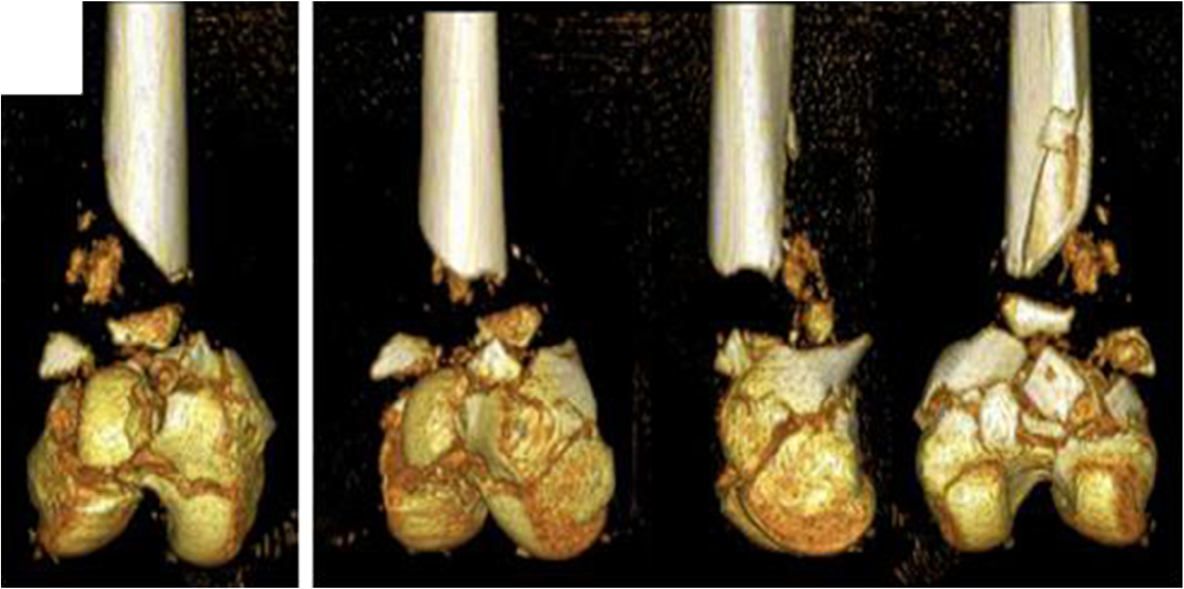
Preoperative imaging (three-dimensional reconstruction computed tomography scan).
